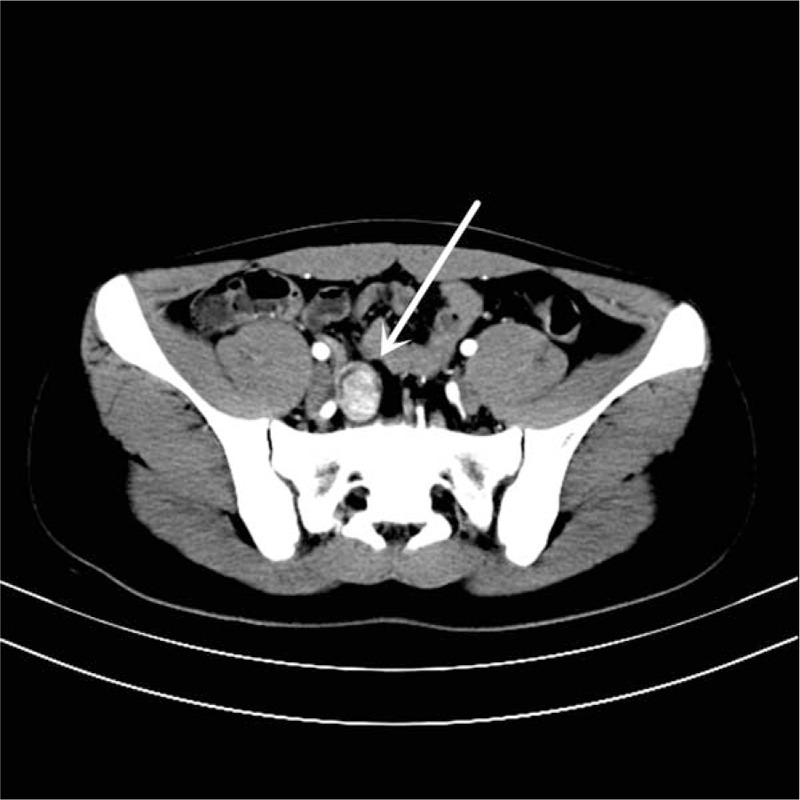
CECT demonstrated a tumor in the right quadrant of the abdomen, size of about 18 × 14 mm in diameter (arrow). CECT = contrast-enhanced computed tomography.
